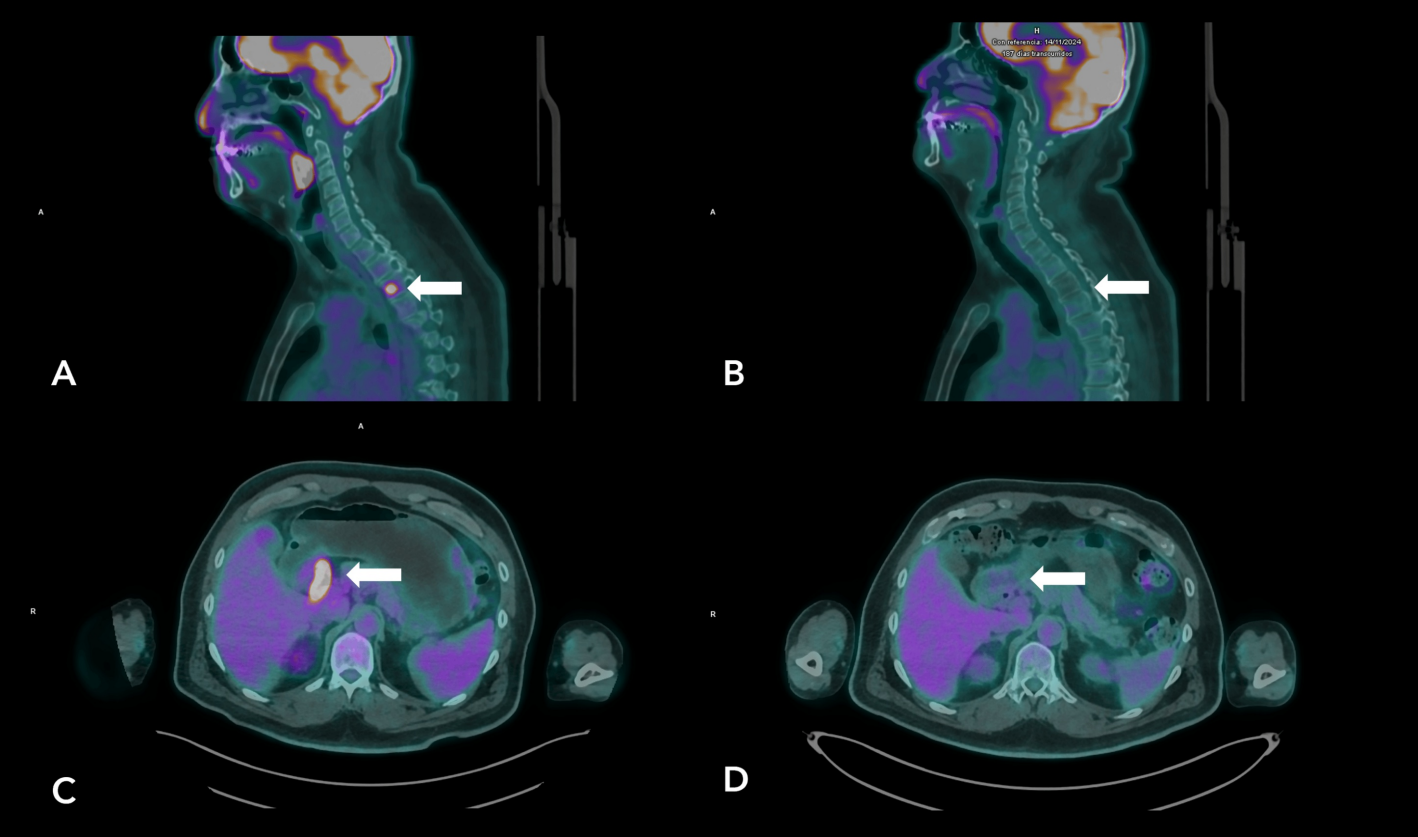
a, b) Sagittal PET/CT fusion images. At staging (a), a hypermetabolic lesion is observed in the T3 vertebral body, with resolution of hypermetabolism following six cycles of R-CHOP (b); the area now demonstrates hypometabolism. c, d) Axial PET/CT fusion images. At staging (c), a hypermetabolic lesion is identified in the first portion of the duodenum, with resolution of hypermetabolism after six cycles of R-CHOP (d); the activity is now within physiological levels.
